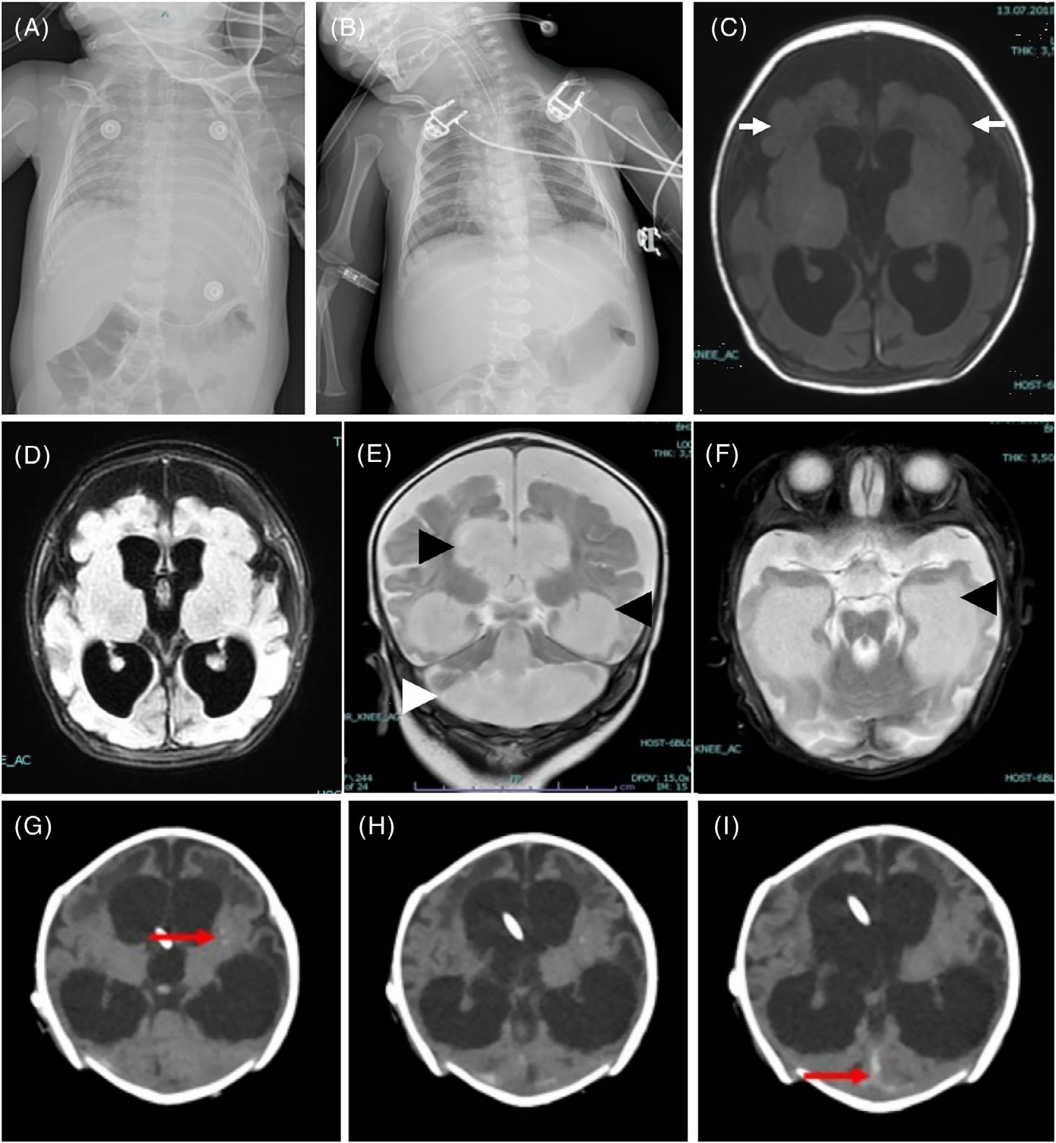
Imaging from Case 2. Chest X‐rays before (A) and after (B) initiation of ventilatory support. (A) Bilateral atelectasis with hypoventilation. (B) Ventilatory support with tracheostomy in situ, bilaterally ascended diaphragms suggesting diaphragmatic dysfunction. Brain MRI T1 (C), FLAIR (D) and T2 (E, F) sequences showing generalised paucity of cortical folding (C, white arrows), hydrocephalus with volume loss of cerebral parenchyma (E, F, black arrows), cerebellar vermis hypoplasia and mega cisterna magna (E, white arrowhead) at 3 months of age. Brain computed tomography (G–I) showing basal ganglia calcifications (G, read arrow) and venous sinus thrombosis (I, red arrow) with derivation of the hydrocephalus.
